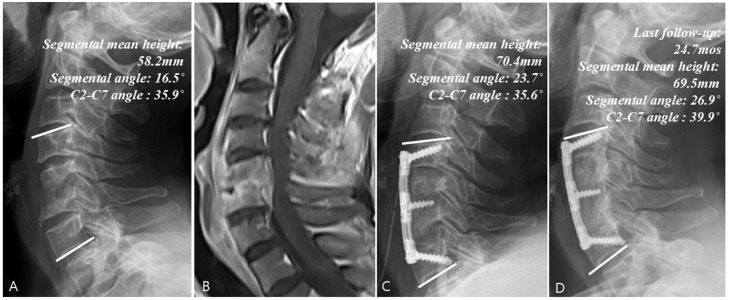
Example of a surgical treatment case. (A) Simple radiographs of a 50-year-old female patient showing C5–C6 vertebrae collapse. (B) Gadolinium-enhanced MR image showing infectious spondylodiscitis at C5–C6, accompanied by prevertebral and epidural abscess. (C) Immediate postoperative plain radiographs showing the state of C5–C6 corpectomy and reconstruction with plate and iliac auto bone graft. (D) Last follow-up (24.7 months) plain radiographs showing stable cervical spine alignment.
